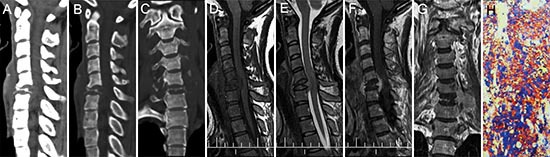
pPNET in a 26-year-old woman CT imaging showed compression fractures in the 6th cervical vertebra (A-C). Precontrast MRI showed an irregular mass around the compressed 6th cervical vertebra that was iso-intense on T1WI (D) and iso-intense on T2WI (E). Contrast MRI showed the mass had significant enhancement (F). Coronal images of the enhanced MRI showed invasion into the paraspinal soft tissues (G). The poorly differentiated small round tumor cells were CD99 positive (H × 100).
